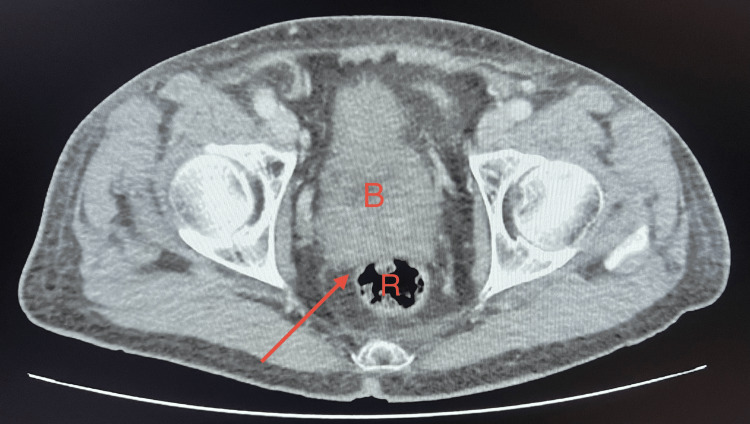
Contrast-enhanced abdominopelvic CT scan (axial pelvic view) Contrast-enhanced abdominopelvic CT scan (portal venous phase), axial pelvic view at the level of the rectovesical pouch, showing no pelvic collection, perirectal abscess, or dependent free fluid. The arrow shows the rectovesical space. B=Bladder, R: Rectum. Informed consent was given for the publication of the study.
